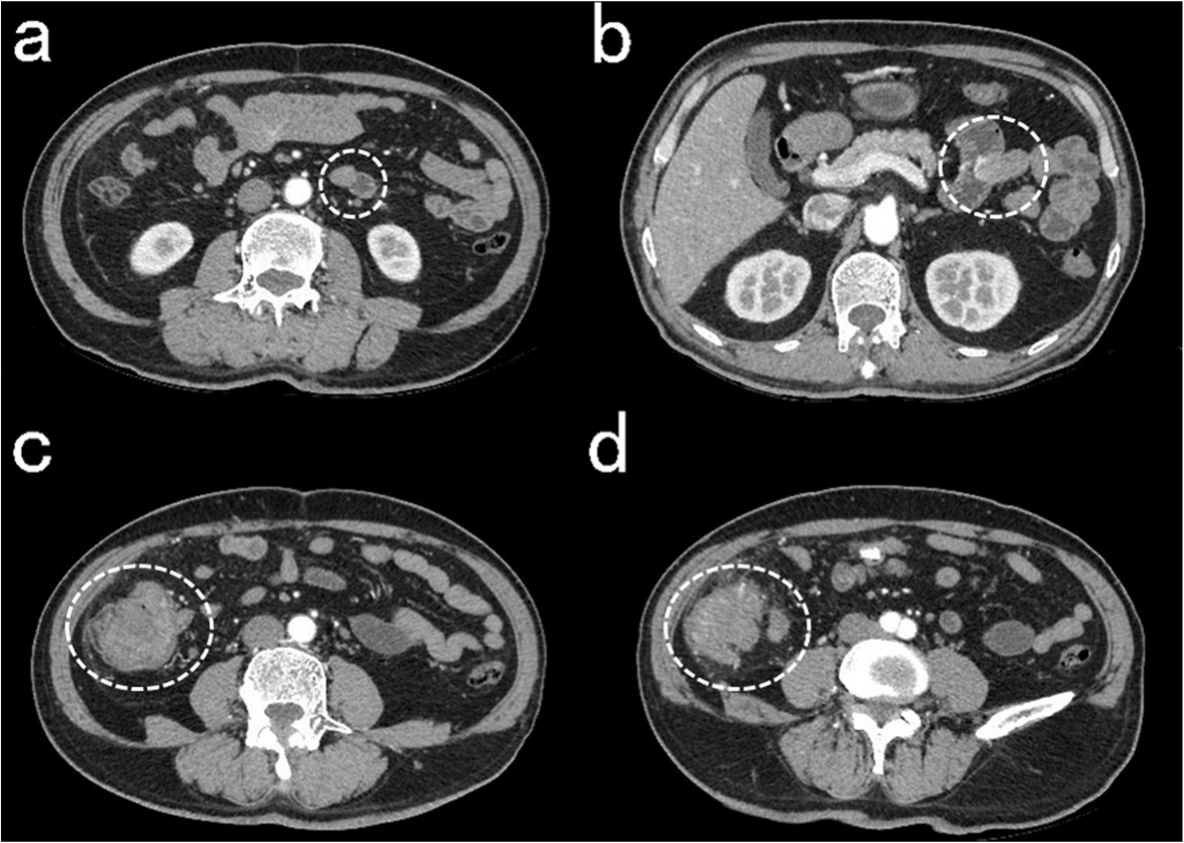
CT (a, b) Jejunal GISTs, c, d Ascending colon cancer
